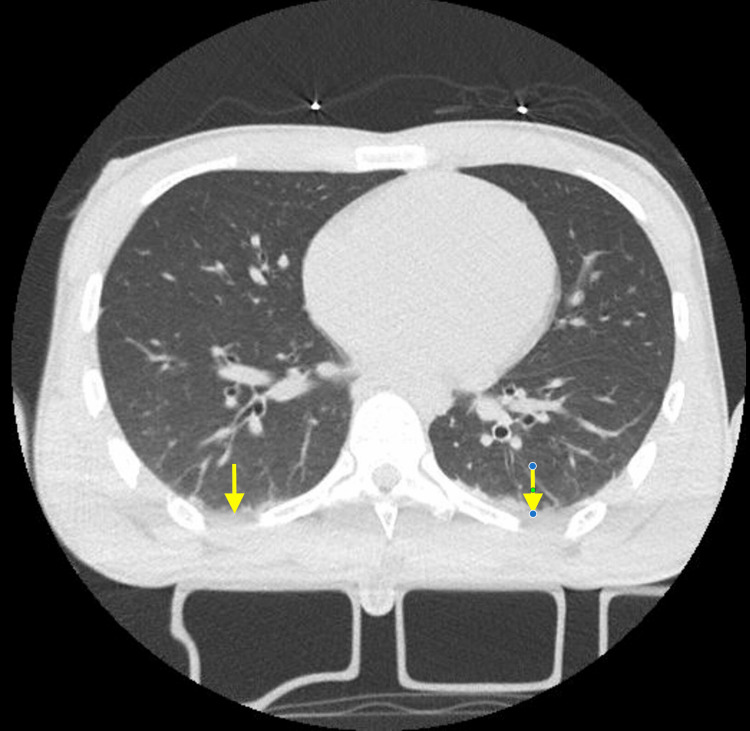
Chest CT scan of the second patient (LB) This axial chest CT image demonstrates a lung window view at the level of the lower thorax, showing mild bilateral base pleural effusion and atelectasis (yellow arrows). There is also a normal pulmonary parenchyma without evidence of consolidation, ground-glass opacities, or masses. The cardiac area is fully visualized without signs of cardiomegaly. CT: computed tomography
